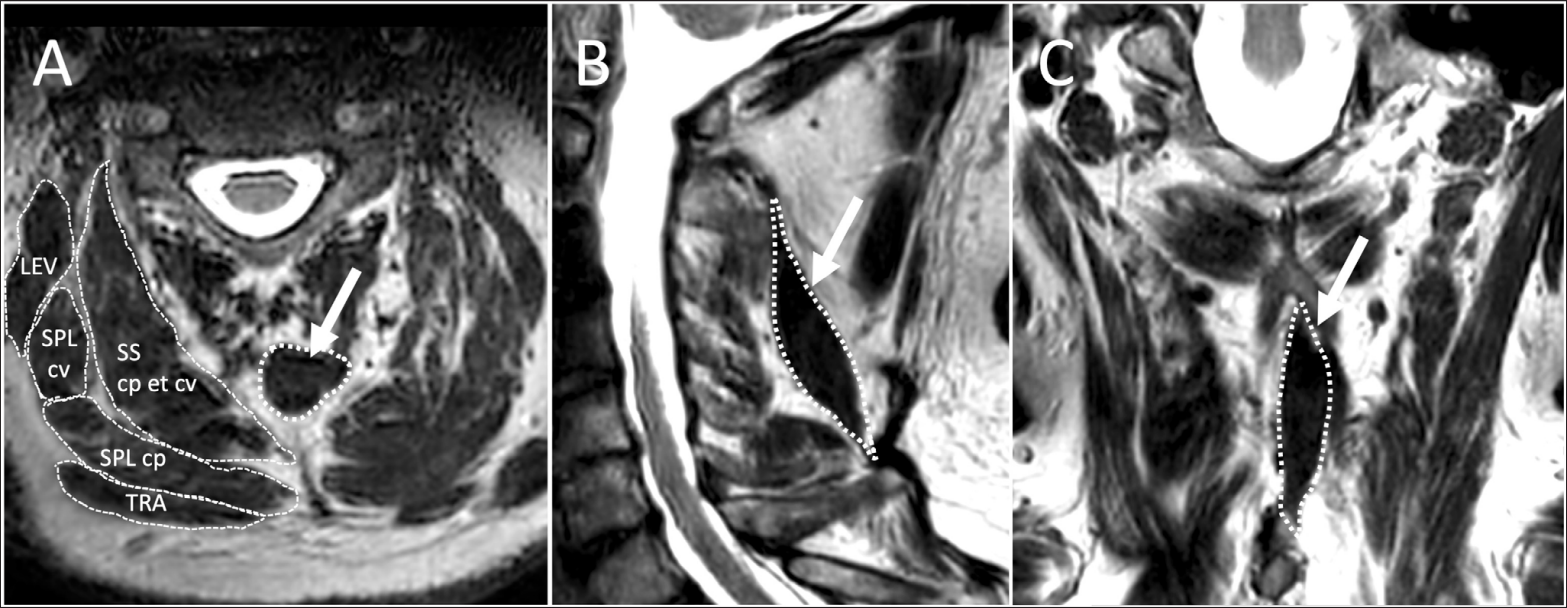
Neck MRI of a subject with retrocollis. T2-weighted images depicting the spinalis cervicis muscle (white encircled, arrow); (A) Axial and (C) coronal images showing the left sided unilateral anlage; (B) sagittal image demonstrating its extension from C7 to C2. SScp et cv = semispinalis capitis et cervicis; SPLcp = splenius capitis; SPLcv = splenius cervicis; LEV = levator scapulae; TRA = trapezius.
